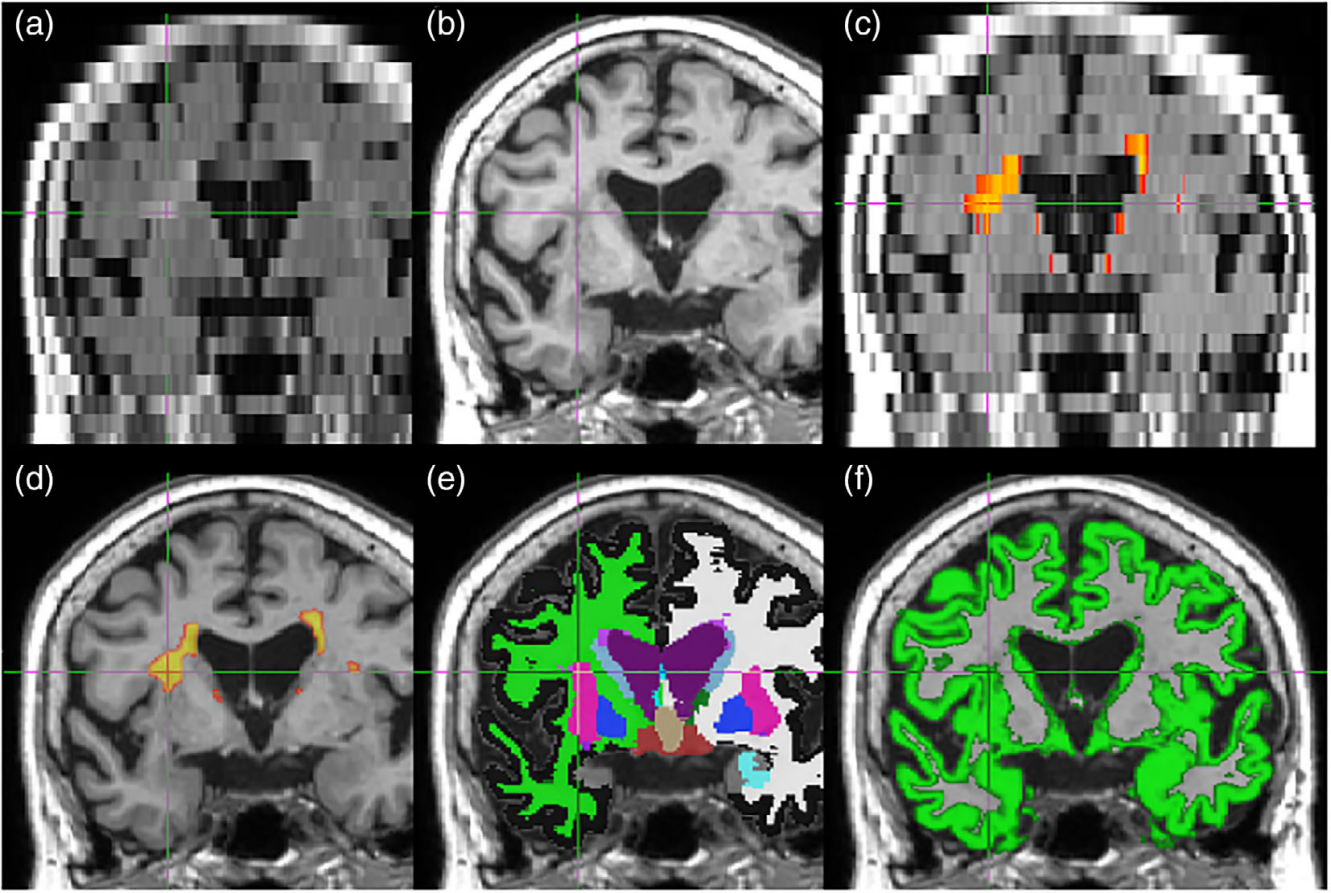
Methods overview. On this coronal slice, a periventricular lesion expands toward the insular cortex. The lesion is visible on the original 2D‐FLAIR image (a), and on the higher‐resolution T1 image (b) although with less contrast. The LST algorithm outcome (c) correctly identified the lesion on the FLAIR image. Our trained ConvNet successfully identified a similar area (d), although at the higher T1 resolution, despite the contrast ambiguity. However, FreeSurfer (e) misclassified part of the lesion as the putamen, while SPM (f) wrongly included it in its gray‐matter mask.
